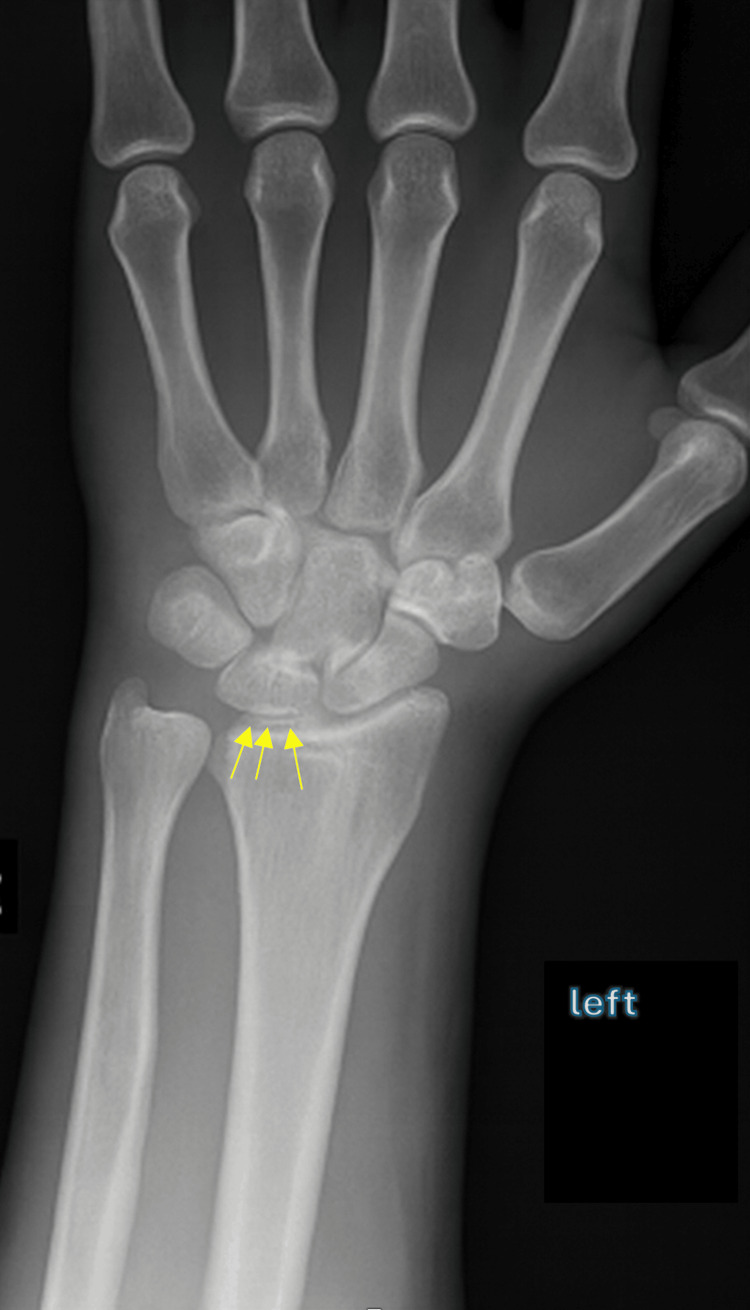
Plain radiograph of the left wrist showing chondrocalcinosis (yellow arrows)
